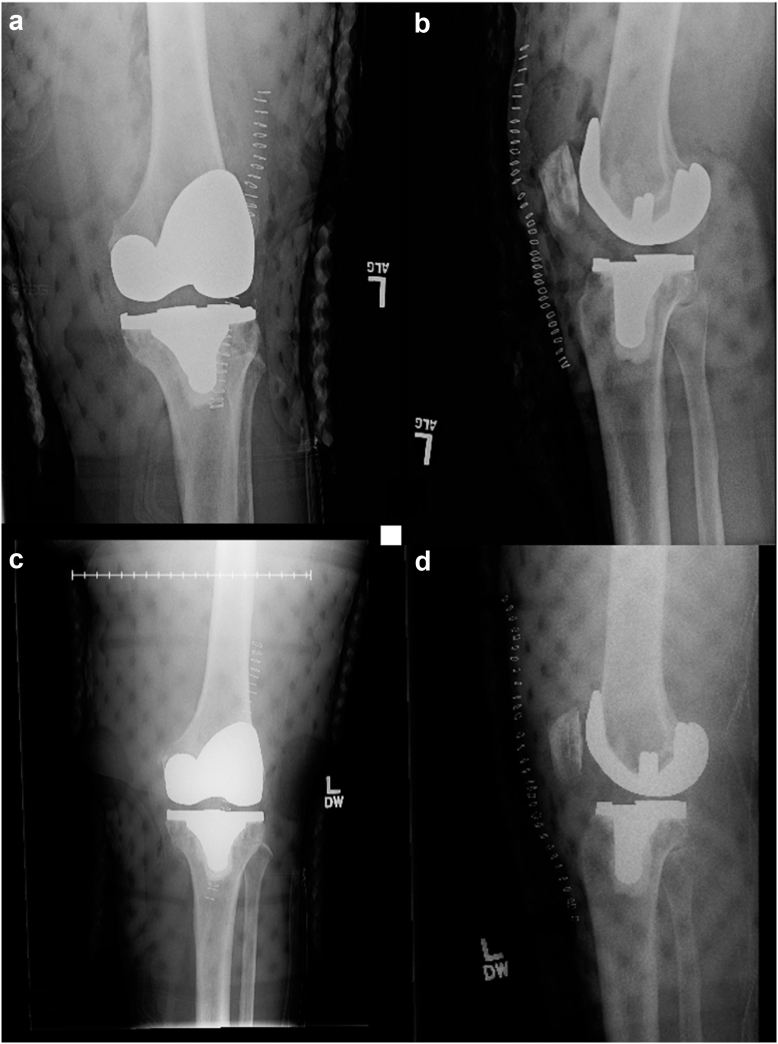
Demonstration of flexion contracture. (a and b) Preoperative anteroposterior and lateral radiographs of a 56-year-old male with a Microport Evolution medial pivot total knee system showing flexion contracture. (c and d) Postoperative anteroposterior and lateral radiographs of the same patient. Intraoperatively, the components were verified as well-fixed, and the polyethylene insert thickness was reduced from a 12-mm cruciate-sacrificing insert to a 10-mm cruciate-sacrificing insert. This modification led to an improvement in flexion contracture. Two years postoperatively, the patient is pain-free with a ROM from 0 to 105°.
